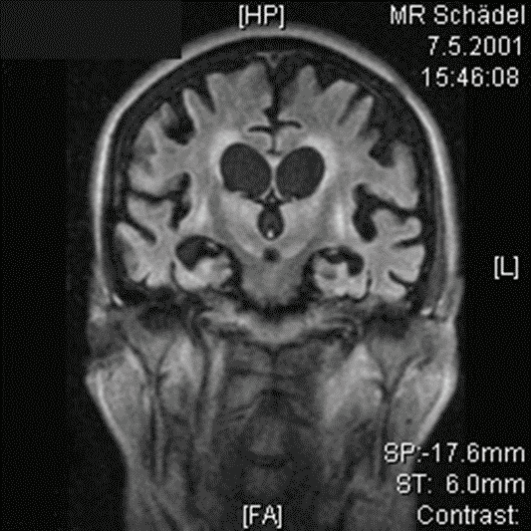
FDG PET scan PVS full stage. Indication: Brain metabolism 10 months after insult. L.D., female, 66 years: PVS full stage following intraoperative cardiac arrest September 2001. Despite immediate resuscitation severe cerebral hypoxemia, massive dehydratation and electrolyte imbalance; postoperative coma. Begin of early neurosurgical rehabilitation 2001/04/25. MRT of the brain (2001.05.07): Massive cortical and subcortical brain atrophy with dilated ventricles (normal intracranial pressure) following cardiac arrest and resuscitation.
